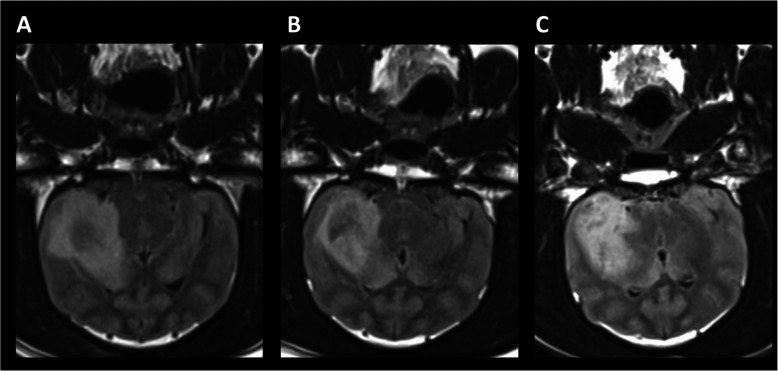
P4 T2 FLAIR at a 1 month pre-treatment, b 1 month post-treatment and c 6 months post-treatment
